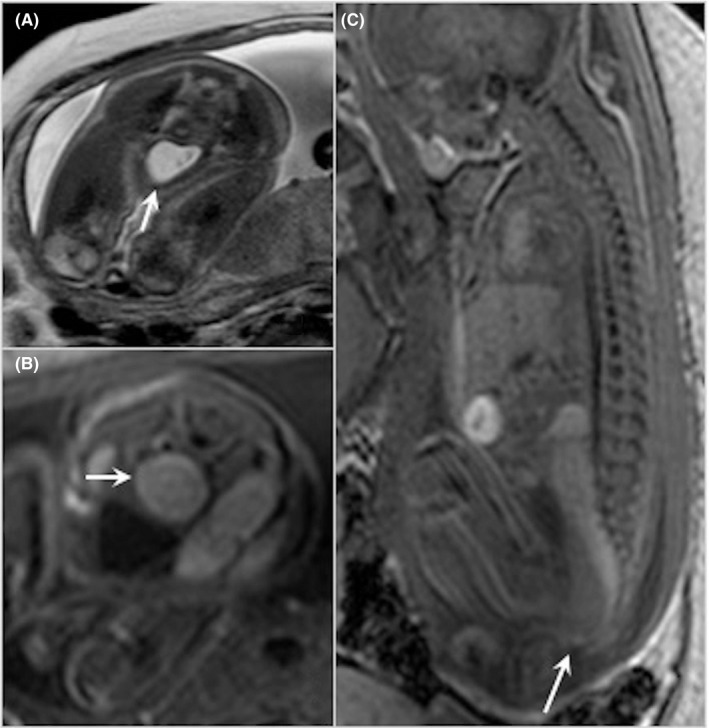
Fetal MRI performed at 31 wk of GA. A, axial T2‐weighted image showing a normal hypersignal bladder (arrow); B, axial T1‐weighted image showing the normal hypersignal rectosigmoid loop (arrow); C, sagittal T1‐weighted image depicting the normal distal end of the rectum to the anal line (arrow)
